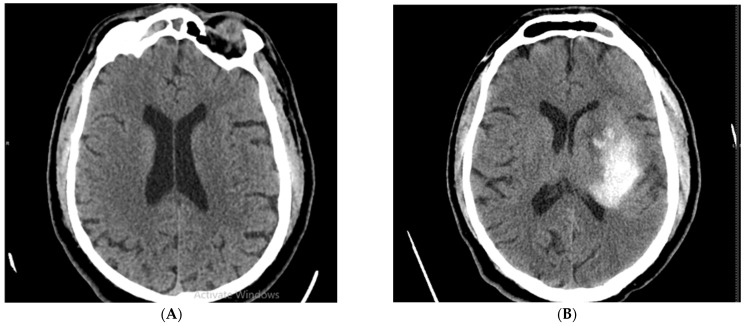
(A) Cerebral CT performed at admission in ED and (B) Hemorrhagic transformation on Cerebral CT performed at 24 h after performing thrombolytic therapy at the same patient.
